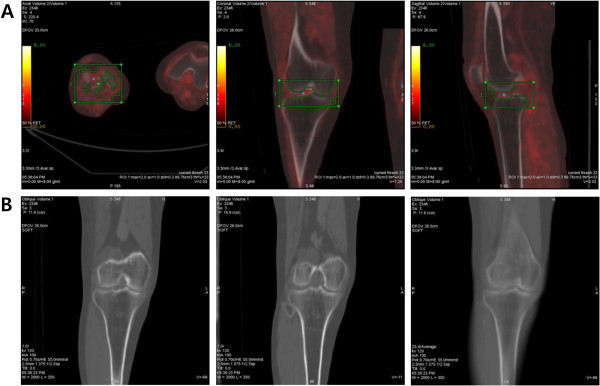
Joint-SUVmean was measured at the ROIs indicated by the green boxes, which included the minimal area between the epiphyseal plates of the femur and tibia in the trans-axial, coronal, and sagittal planes. The blue rectangles indicate the points of the highest SUV of intra-articular space, the ROIs for intra-articular SUVmax are precisely delineated by crooked lines within the green box for joint-SUVmean. The point of intra-articular SUVmax was confirmed in trans-axial, coronal, and sagittal slices (A). Coronal-reconstruction CT images were arranged in the anterior-posterior view of knees for K/L grading (B).
